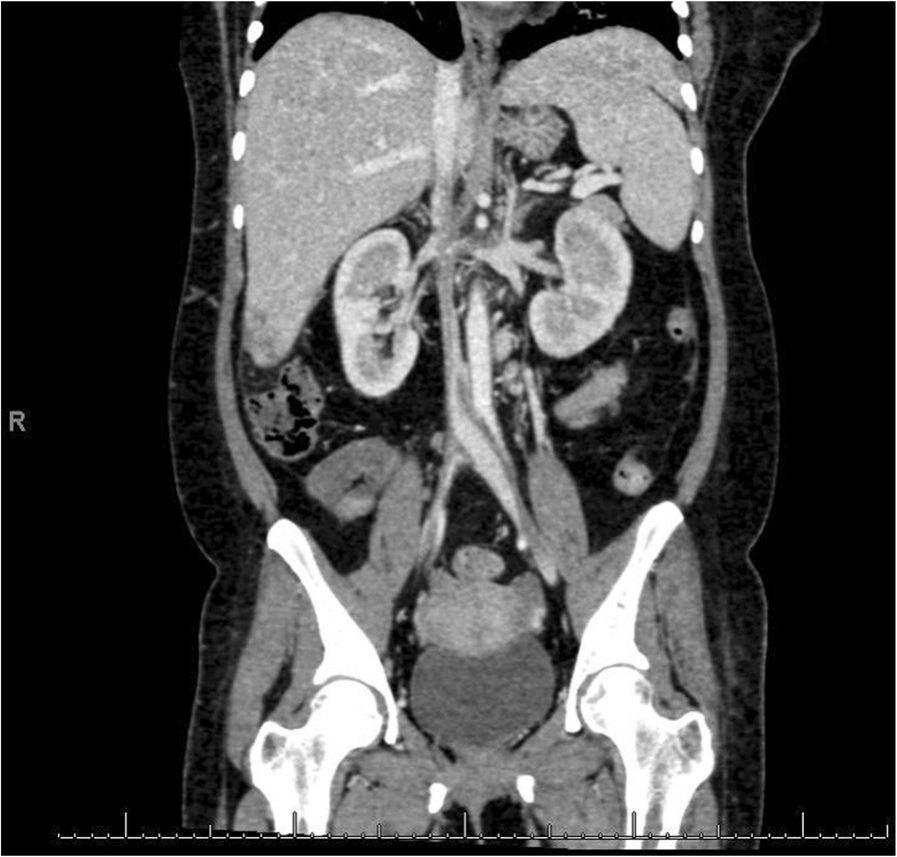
Computed tomography scan showing the extent of the inferior vena cava thrombus to the iliac and the distal left renal vein.
